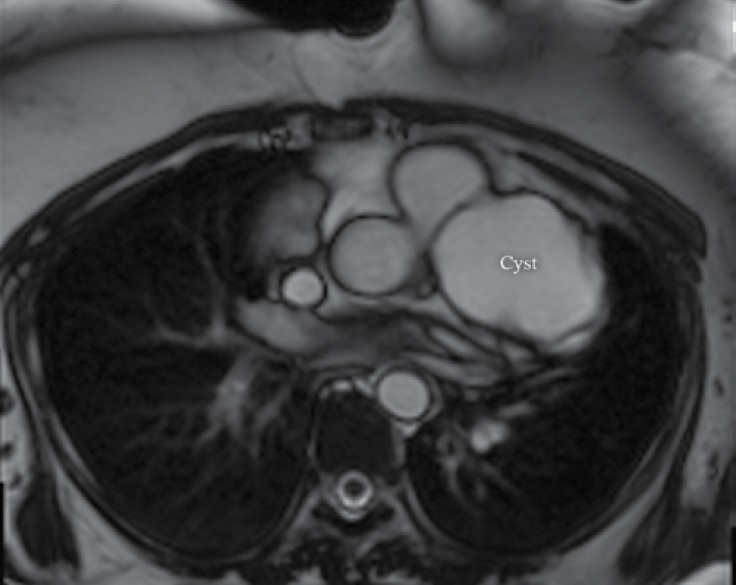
Cardiac magnetic resonance imaging shows a cyst in the patient’s mediastinum.
